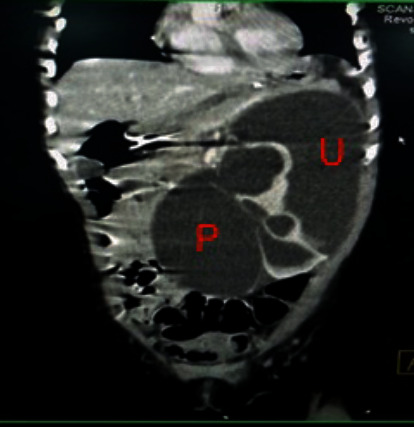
CT showing severe PUJO, ruptured with compressive urinoma. P: pelvis, U: compressive urinoma.
